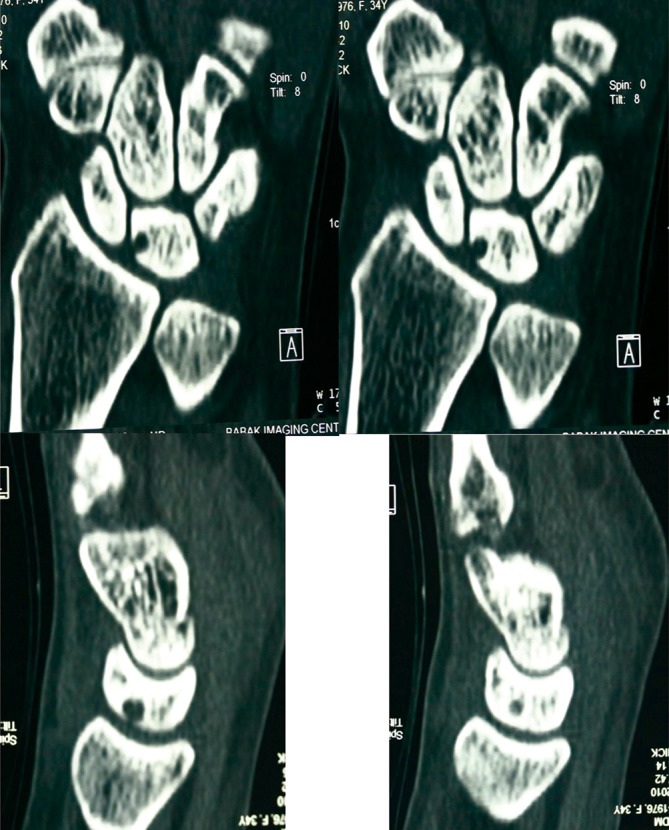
CT scan view of the ganglion cyst
